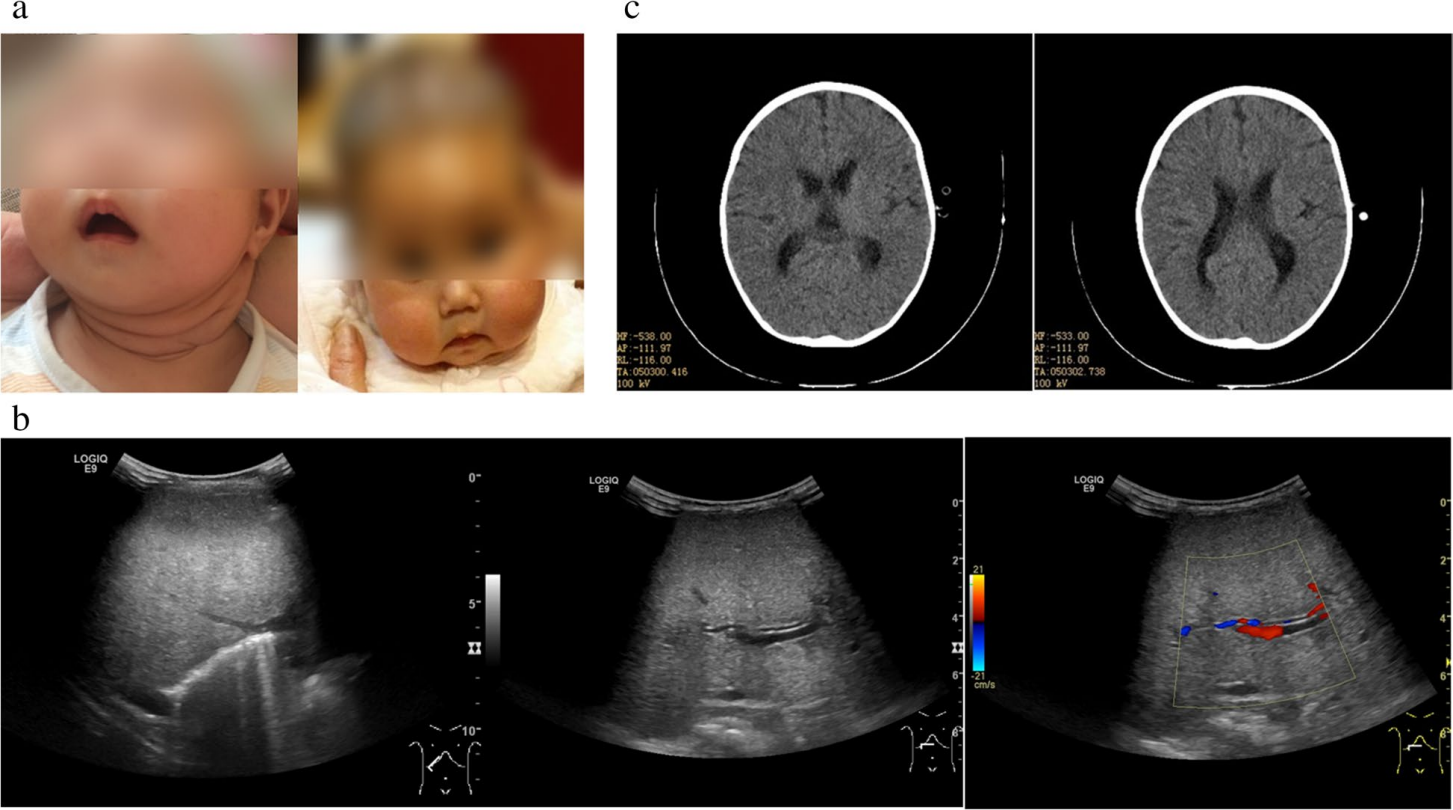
Clinical manifestations. a Face feature. This girl had abnormal fat, round face, and soft translucent skin. b Ultrasound examination. Conventional B-mode ultrasound (left) showed that the liver was significantly enlarged and the echo of the liver parenchyma was coarse, suggesting the possibility of cirrhosis. The B-mode ultrasound (middle) and color Doppler flow imaging (CDFI)(right) showed enlargement of hepatic artery (arrows). c CT scan of brain. The boundary between gray matter and white matter was not clear, the sulci and cistern were slightly widened and deepened, the left lateral ventricle was slightly widened, about 1.0 cm(arrows)
